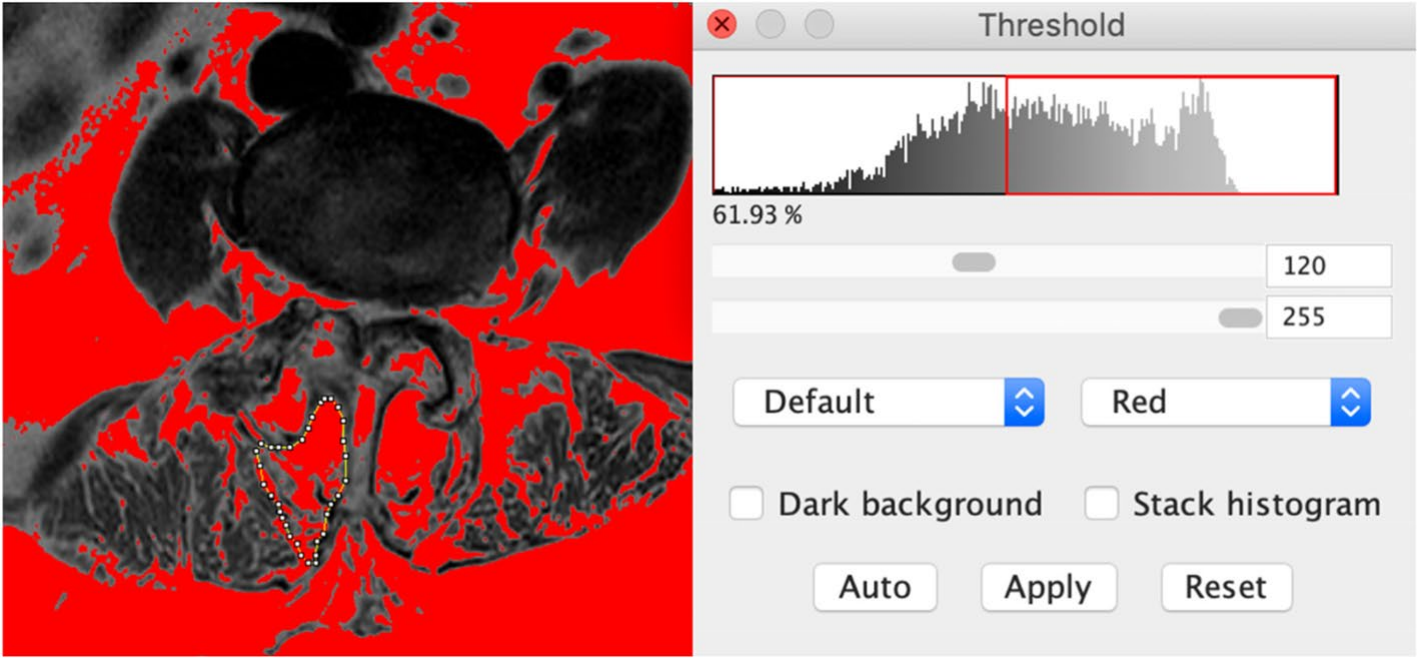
Measurement method of the multifidus muscle fatty infiltration. The Image J Threshold method on the middle layer of magnetic resonance image in each segment
